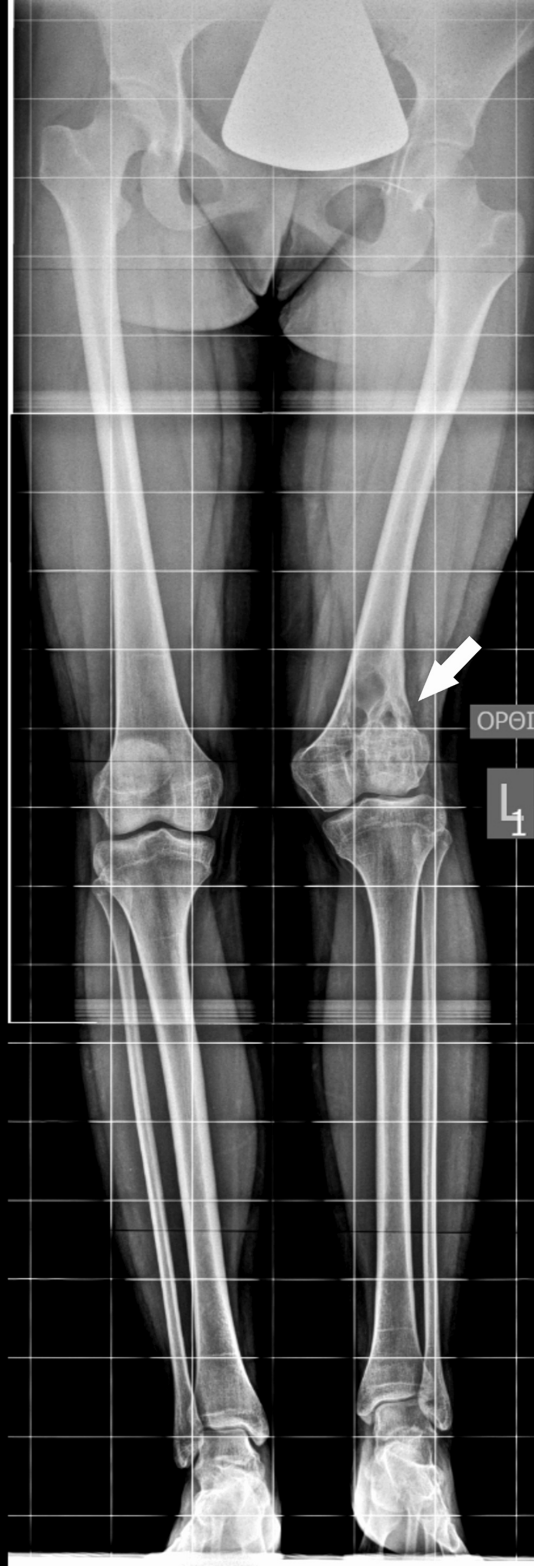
A standing X-ray taken at the end of growth, depicting valgus deformity of the knee and LLD. LLD: Leg length discrepancy.
